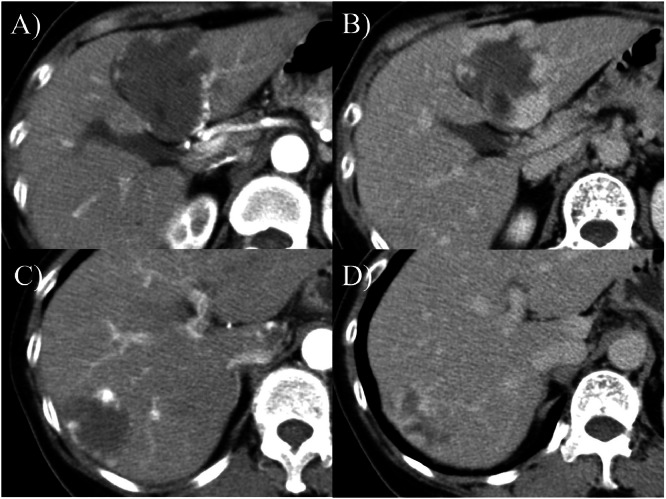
Dynamic abdominal computed tomography findings (2006+5 years): Masses of 58×47 mm in liver S2/3 (A, B) and 34×27 mm in liver S8 (C, D) are observed. In the arterial phase, peripheral nodular enhancement is observed (A, C). In the delayed phase, progressive centripetal filling-in is observed (B, D).
